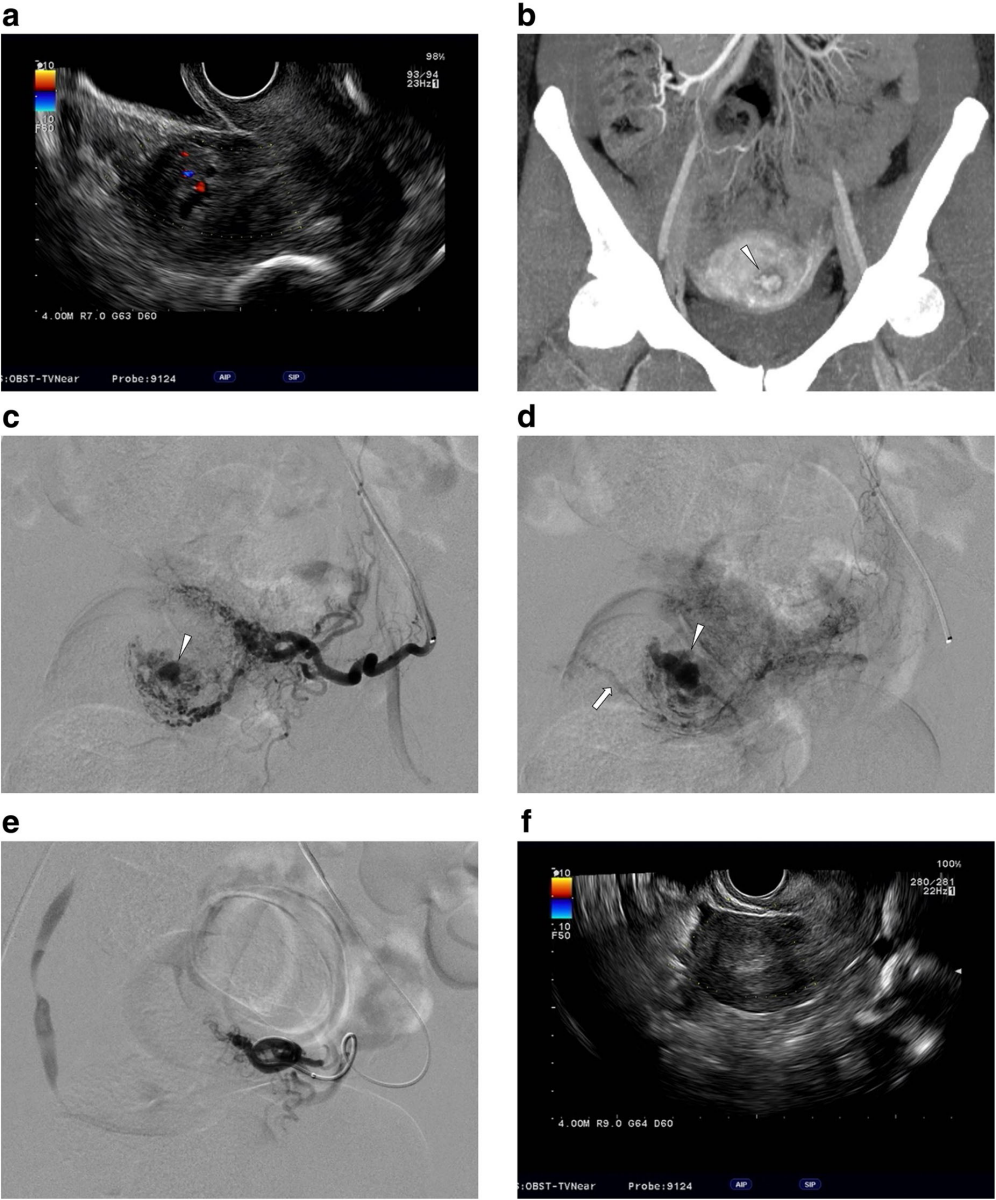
A 33-year-old woman with vaginal bleeding caused by retained placenta. a. Color doppler TVUS showed vascularity (arrowhead) in the uteroplacental tissue. b. Coronal MIP image of arterial phase CE-CT showed intrauterine vascular mass (arrowhead) fed by left uterine artery. c. Left uterine arteriogram showed dilated vascular channel (arrowhead) in arterial phase uterine arteriography. d. Venous drainage (arrow) from dilated vascular channel (arrowhead) depicted in the capillary phase. Drainage vein connected to right uterine vein in the venous phase. Microcatheter was inserted into the blood sinus feeding artery. Target embolization was performed using GS. e. Post-embolization left uterine arteriography confirmed disappearance of the dilated vascular channel. f. At 2 days after the TAE procedure, the intrauterine hyper vascular lesion disappeared on TVUS. In this case, expectant management after TAE was chosen and no re-bleeding was observed
